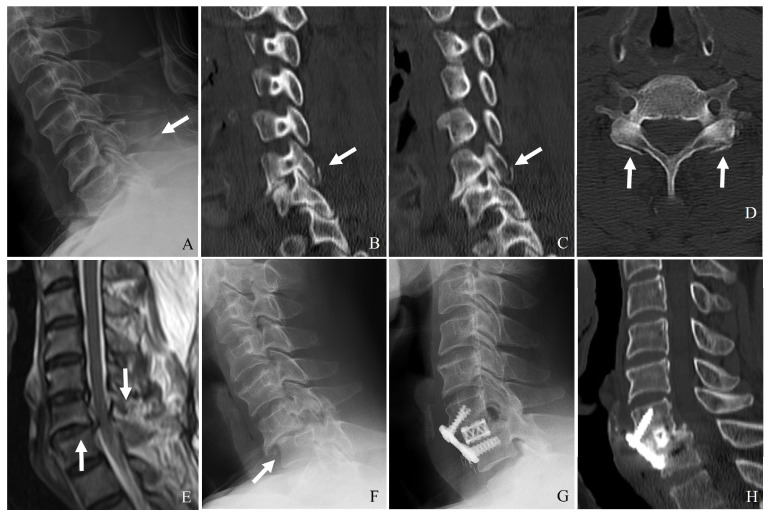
Plain X-ray (A), computed tomography (B–D), and magnetic resonance aging (E) demonstrate the widening of the interspinous distance, bilateral facet fractures, and injuries to the posterior ligamentous complex and posterior longitudinal ligament at C6–C7 (white arrows). Delayed bilateral facet dislocation of C6–C7 occurred 1 month after wearing a rigid brace (white arrows) (E,F). The patient underwent anterior cervical discectomy, open reduction, and fusion of C6–C7 (G,H).
